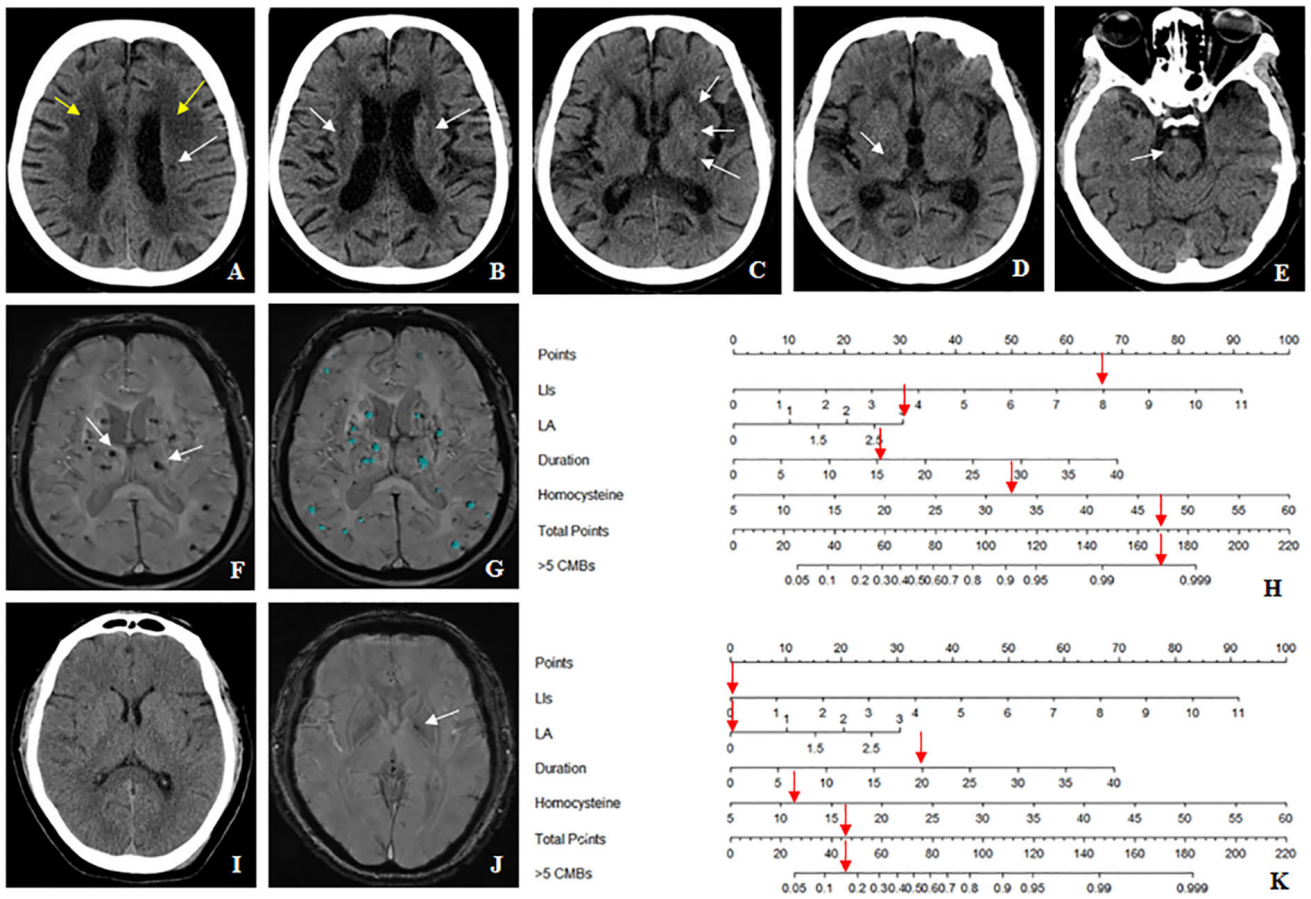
The nomogram accurately differentiates >5 cerebral microbleeds (CMBs) with ≤ 5 CMBs in the hypertensive population. (A–H) Case 1: a 64-years-old female with hypertension for 15 years, whose homocysteine level was 32.9 μmol/L. (A) The Blennow score of the LA was 3 (yellow arrow). (A–E) The number of LIs defined by CT was 8 located in the left basal ganglia, right thalamus, bilateral semiovale center, and brainstem region (white arrow). (F,G) susceptibility-weighted imaging (SWI) shows multiple CMBs (>5) (white arrow), which were diagnosed by BioMind AI software (green marker). (H) According to the combined model, the prediction probability of >5 CMBs was more than 99% (red arrow). (I–K) Case2: a 54-years-old male with hypertension for 20 years, whose homocysteine level was 11.1 μmol/L. (I) Both the Blennow score of the LA and the number of LIs were 0. (J) Only one CMB was seen in the left basal ganglia region (white arrow), which met the diagnostic criteria of ≤ 5 CMBs. (K) According to the combined model, the prediction probability of >5 CMBs was between 10 and 20% (red arrow).
